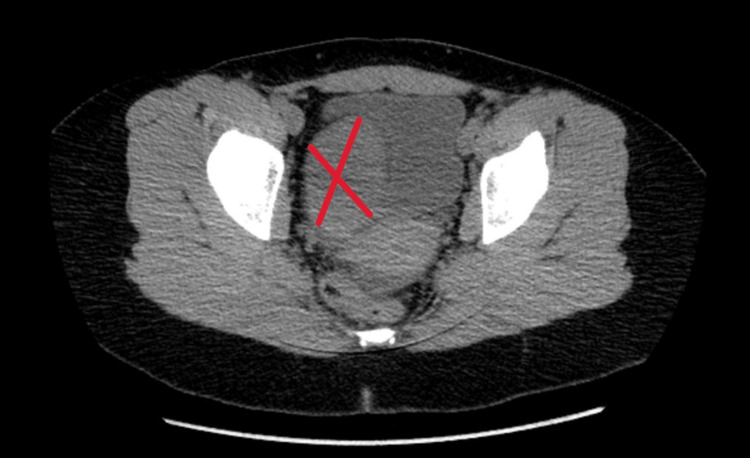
CT scan of the abdomen and pelvis showing a 6.6 x 9.2 x 10.0 cm adnexal mass (red cross). This mass is distinguished from the surrounding tissue by its size, shape, and the absence of typical ovarian follicular structure, suggesting the presence of a complex solid-cystic lesion
